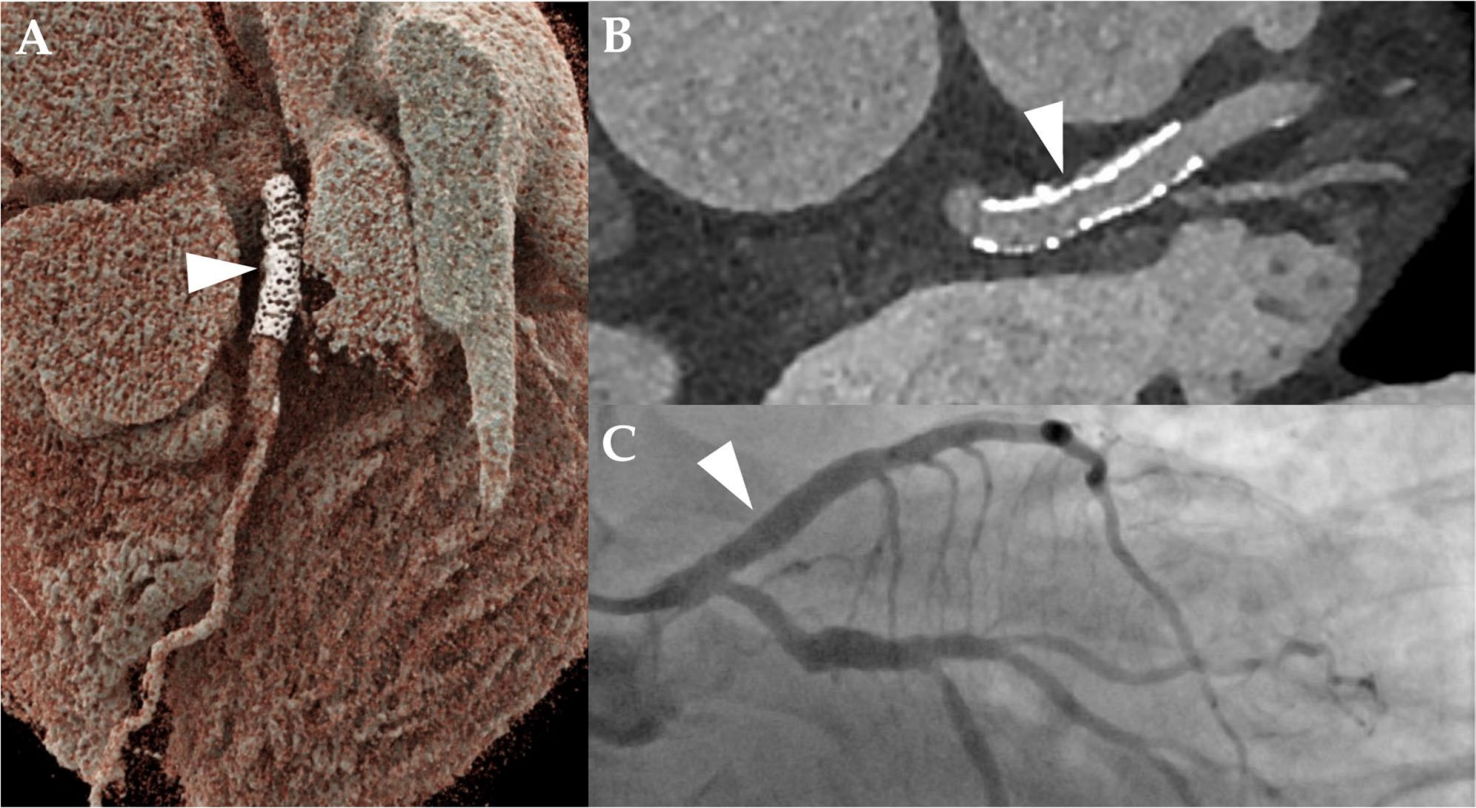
Ultra-high-resolution photon-counting detector CT angiography (PCD-CTA) of the heart of a 69-year-old male patient with severe aortic valve stenosis and a history of coronary stent implantation in the distal left main and proximal left anterior descending artery (LAD) (arrowhead in A, B, and C). A Three-dimensional cinematic rendering of the heart, showing the stent in the LAD. B Curved multiplanar reformation showing the absence of in-stent stenosis. The stent was rated as “excellent”—Likert 1 by one reader—and as “good”—Likert 2 by the other reader. C Invasive coronary angiography at caudal 30° view, verifying stent patency
